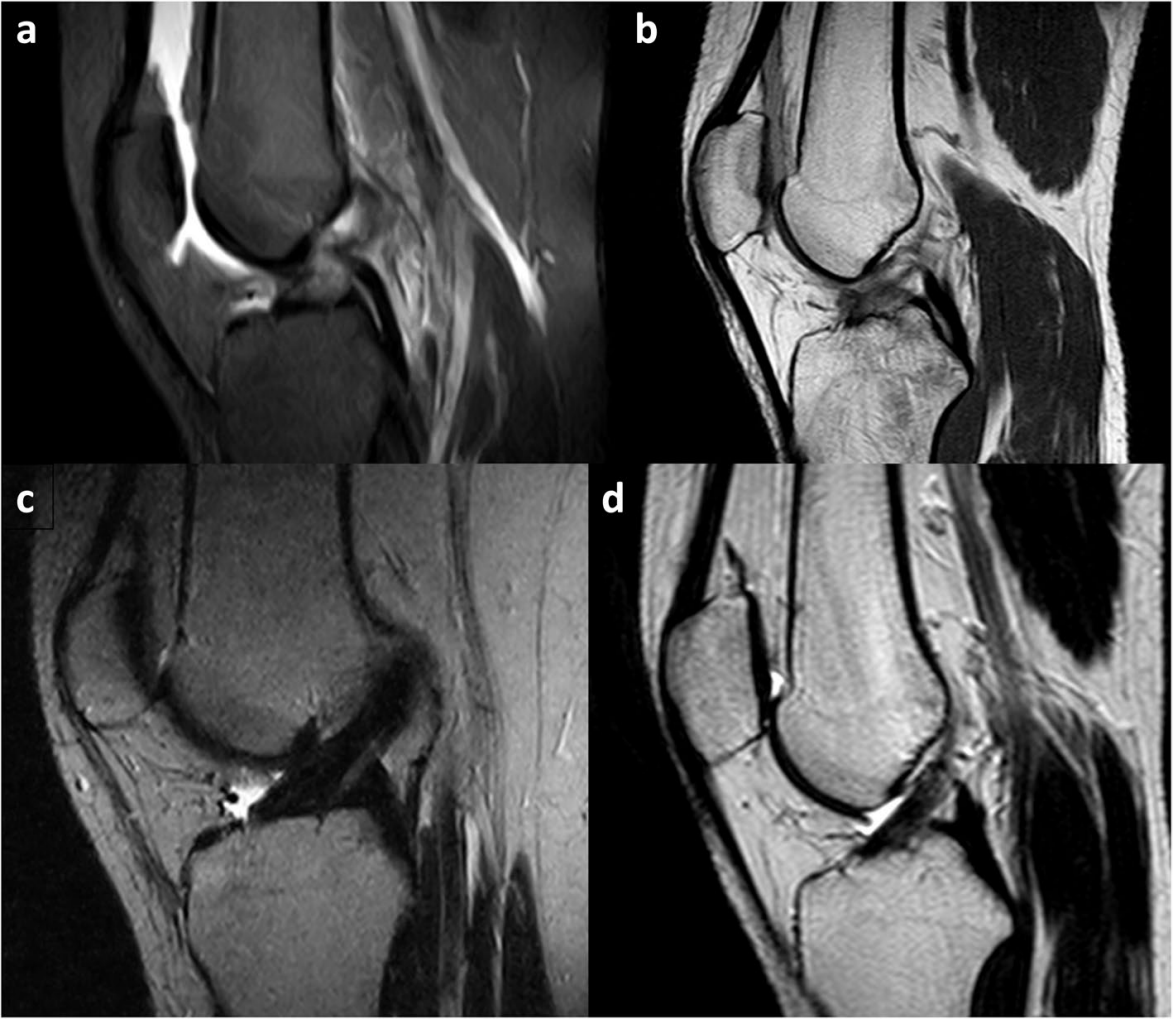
MRI appearance of patient 1 and patient 3 at the time of diagnosis and six months later, a, First MRI of patient 1 after knee injury in a short tau inversion recovery (STIR) sequence and, b, first MRI of patient 3 in a T2 weighted sequence, showing both swelling, complete discontinuity and increased signal of ACL. c, and d, MRI appearance in T2 weighted sequence of patients 1 and 3, respectively, showing complete recovery of signal intensity and continuity of anterior cruciate ligament.
